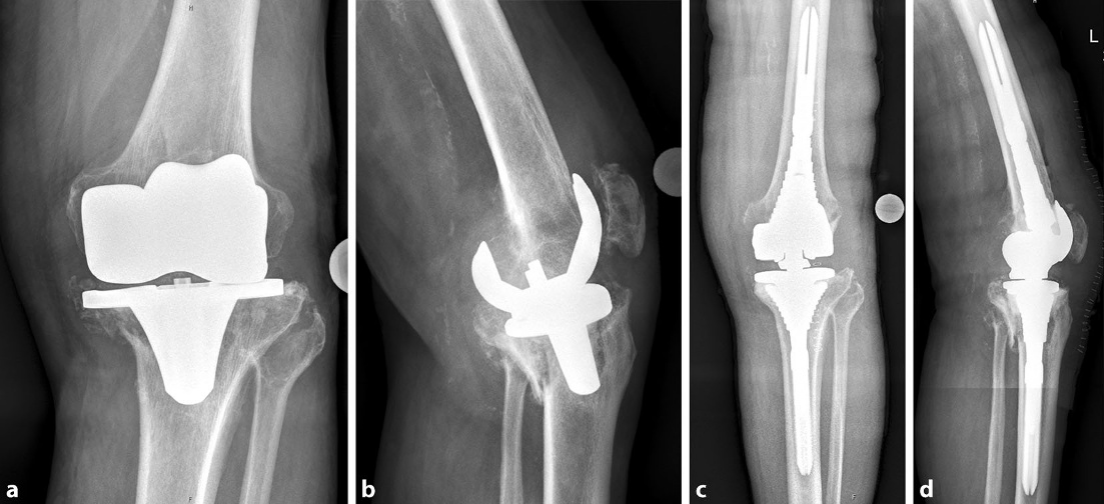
X‑ray radiographs (a anteroposterior and d lateral view) of a patient with aseptic KTEP loosening and simultaneous tibial and femoral periprosthetic fractures with metaphyseal bone loss after explantation of the primary components. Significant osteoporosis was identified during surgery (courtesy of the Department of Diagnostic and Interventional Radiology, University Hospital of Leipzig, all rights reserved). c, d  The same patient (c anteroposterior, b lateral view) after removal of the loosened total knee components and implantation of a rotating hinge knee revision system with metaphyseal sleeves (courtesy of the Department of Diagnostic and Interventional Radiology, University Hospital of Leipzig, all rights reserved)
